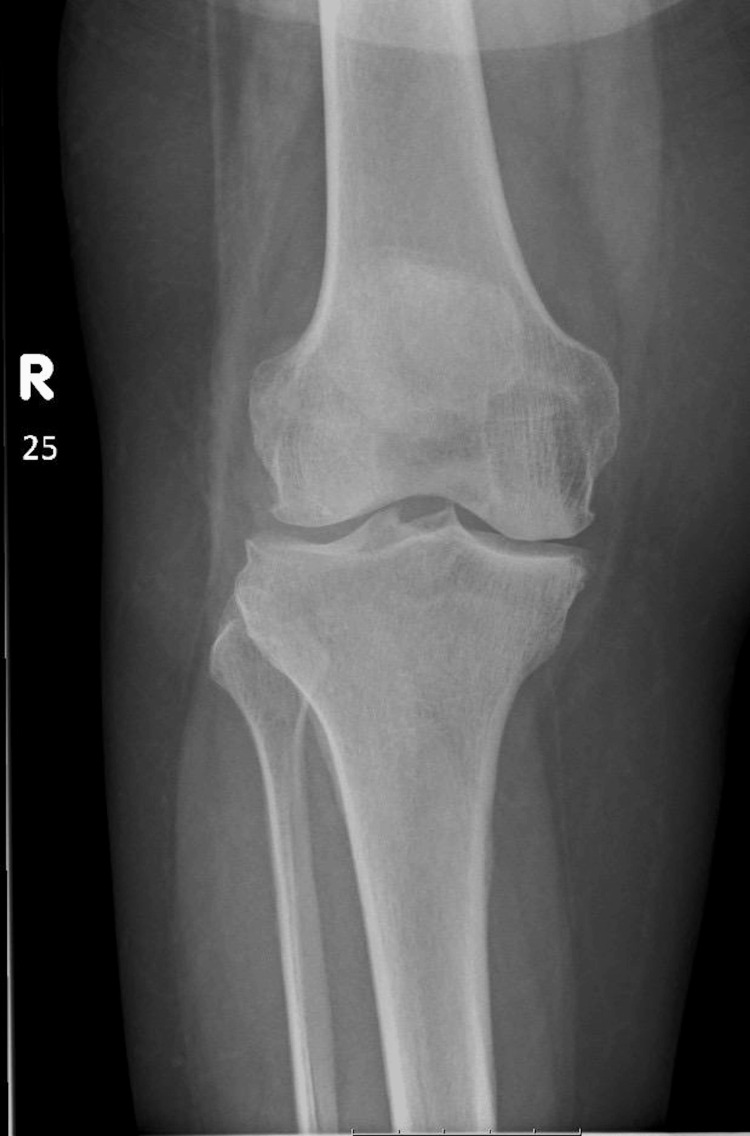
Representative radiograph of Kellgren-Lawrence grade 3 knee osteoarthritis Anteroposterior weight-bearing knee radiograph demonstrates moderate osteoarthritis with definite joint space narrowing, multiple osteophytes, and early subchondral sclerosis. Findings are consistent with Kellgren-Lawrence grade 3 changes.
